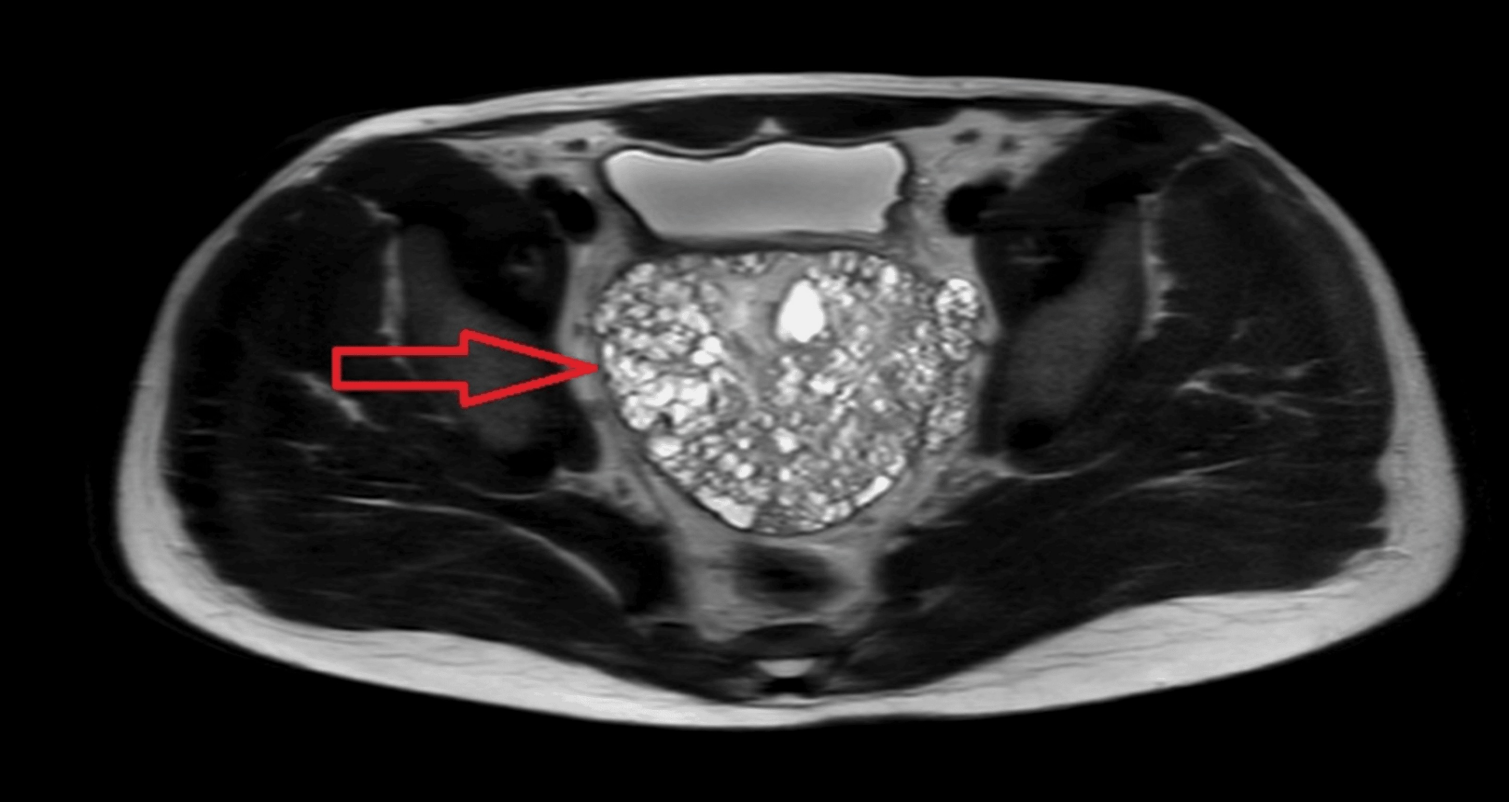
Abdominal MRI scan using T2-weighted turbo spin echo in the transverse plane showing a large, multicompartment, microcystic pelvic mass (101 x 75 x 86 mm) (arrow).
